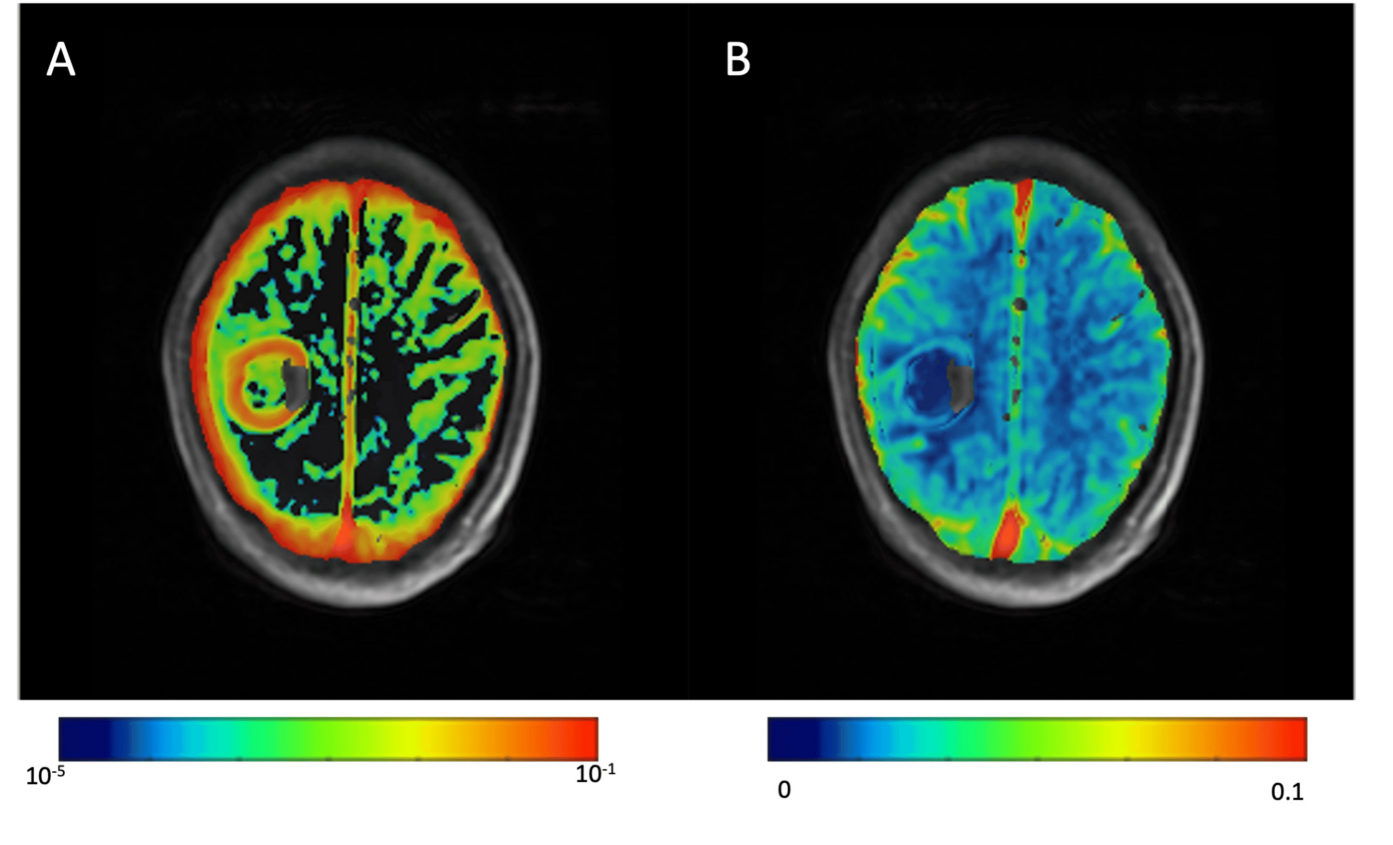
Gadolinium-based contrast DCE-MRI vascular permeability maps (A) An increase in blood-brain barrier leakage rate (Ktrans); (B) blood plasma volume (Vp) in the peri-ablated area was low or absent DCE-MRI: dynamic contrast-enhanced magnetic resonance imaging
